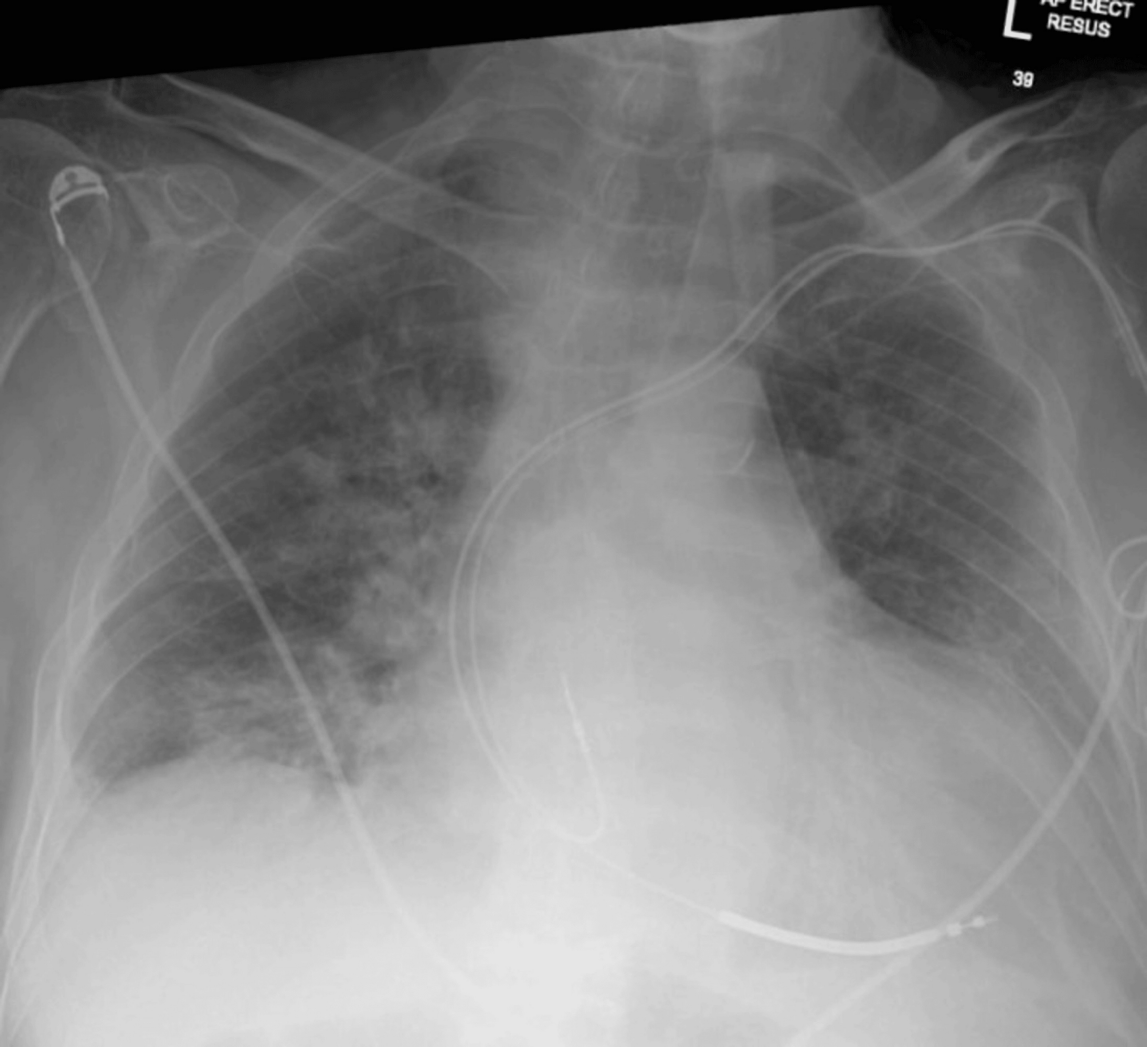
Portable chest X-ray(AP view, semi-erect position) on admission showing pulmonary congestion and cardiomegaly
